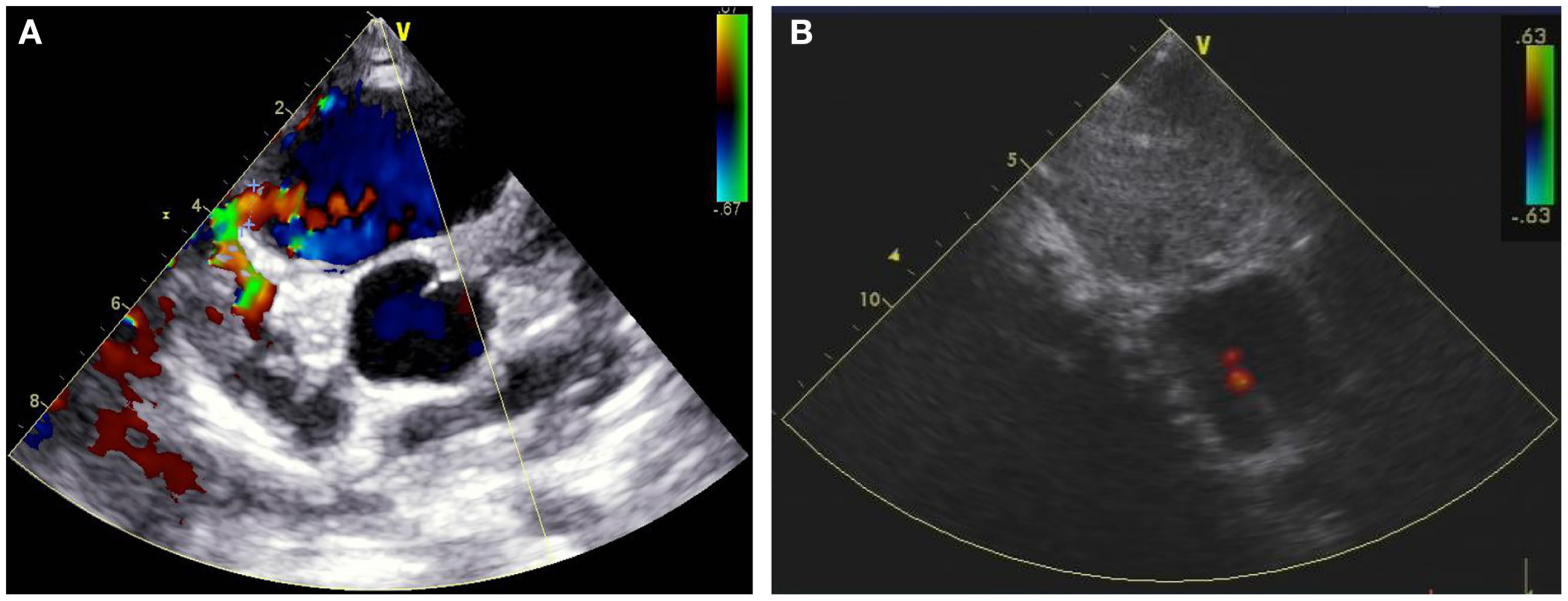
Transthoracic echocardiography showing that a 6.28-mm coronary fistula into the right ventricle (A, arrow) was detected preoperatively, and undetectable 1 week post-surgical ligation (B).
